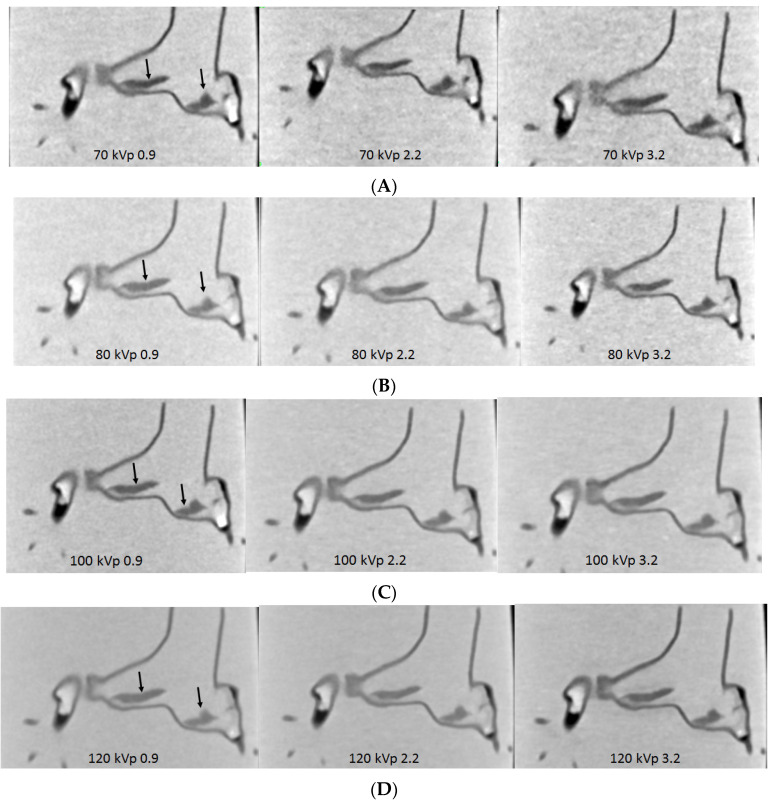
Computed tomography pulmonary angiography (CTPA) in the detection of main pulmonary embolism with different scanning protocols. (A,B) When pitch was increased to 3.2, image noise was increased with 70 and 80 kVp protocols; (C,D) in contrast, no significant change of image quality was noted with 100 and 120 kVp protocols, regardless of pitch values. Arrows refer to the thrombus in the main pulmonary arteries. Reprinted with permission under open access from Aldosari et al. [83].
